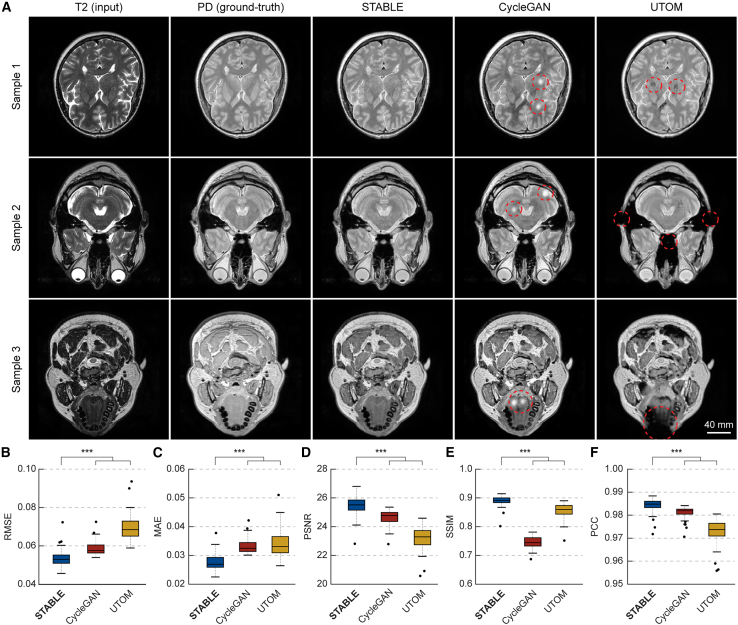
Virtual MRI modality transfer using STABLE (A) STABLE compared with unpaired image-to-image translation methods in translating T2-weighted MRI to PD-weighted MRI data. From left to right: input T2-weighted MRI (left), corresponding GT PD-weighted MRI (second column), followed by the results from STABLE, CycleGAN, and UTOM. Example slices from three different subjects are presented. Red circles highlight areas where CycleGAN and UTOM generate artifacts or fail to capture fine structural details. Scale bar, 44 mm. (B–F) Box-and-whisker plots showing the quantitative comparison between methods across several evaluation metrics: (B) RMSE, (C) MAE, (D) PSNR, (E) SSIM, and (F) PCC. Two-sample t tests were performed (*n* = 64 for each test, representing the number of volumetric MRI samples; n.s. *p* > 0.05, ∗*p* ≤ 0.05, ∗∗*p* < 0.01, and ∗∗∗*p* < 0.001).
